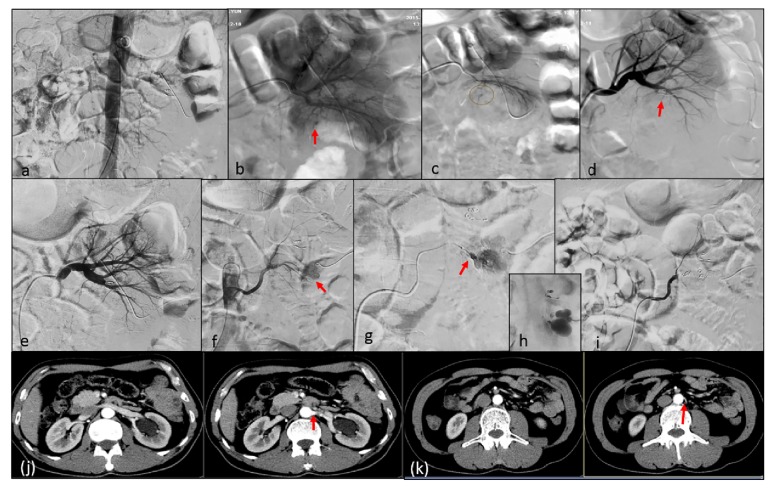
Secondary embolization in a patient with PA after PCNL. (a) Abdominal aorta angiography cannot show the number of left renal artery clearly because of the interference of intestinal gas. (b, c) Selective left renal arteriography shows a PA (red arrow). (d) Angiogram after coil embolization showing complete occlusion of the PA. (e-h) Angiogram 3-day after first time TAE shows a new PA (red arrow) arising from accessory left renal artery, which arises at approximately the inferior level of third Lumbar vertebra. (i) After coil embolization, angiogram shows complete occlusion of the PA. (j, k) The CT image shows the location of left renal artery and accessory left renal artery.
